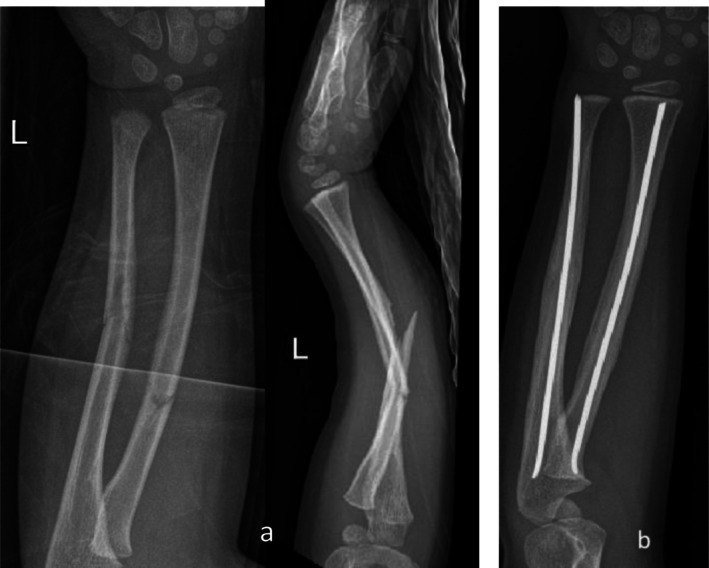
(a) AP and LL view of displaced fracture of the radius and ulna; (b) Postoperative radiograph showing retrograde ESIN fixation and healing.
